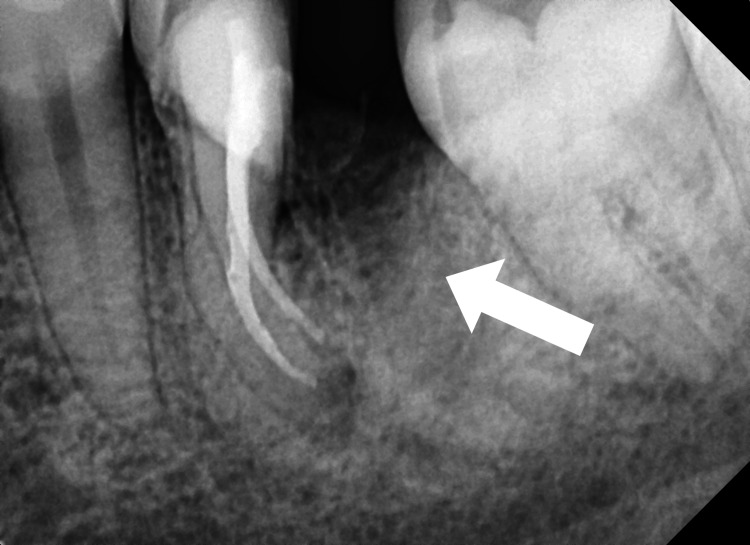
Radiographic follow-up at three months showing the healed extraction socket of the distal root of tooth number 36
